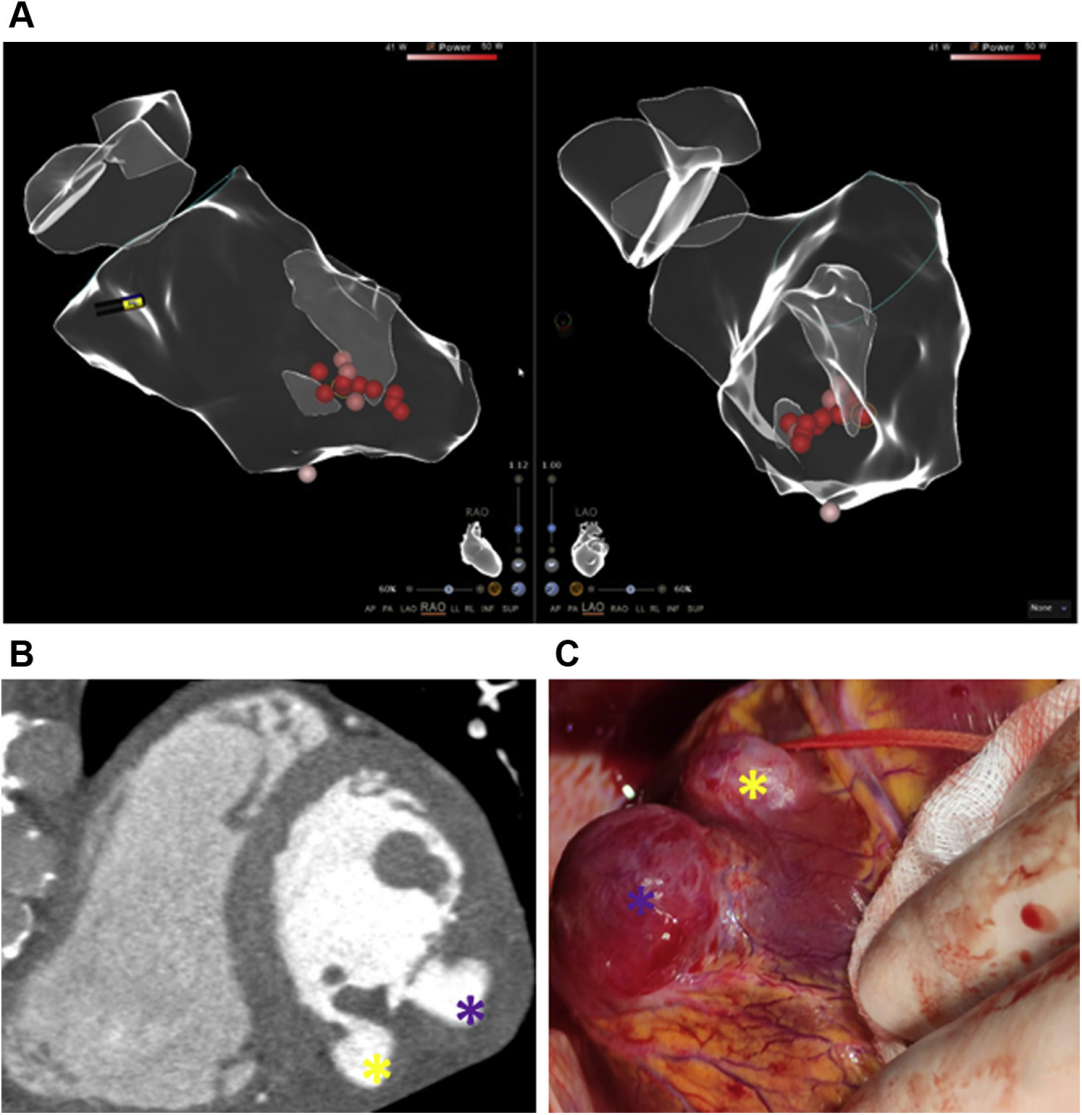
Ablation Dots and Pseudoaneurysms Location (A) Left ventricle anatomical map with right anterior oblique and left anterior oblique projections, showing the 2 areas where radiofrequency was applied. (B) Short-axis CT scan showing inferior pseudoaneurysm (yellow asterisk) and inferolateral pseudoaneurysm (purple asterisk). (C) Surgical view.
